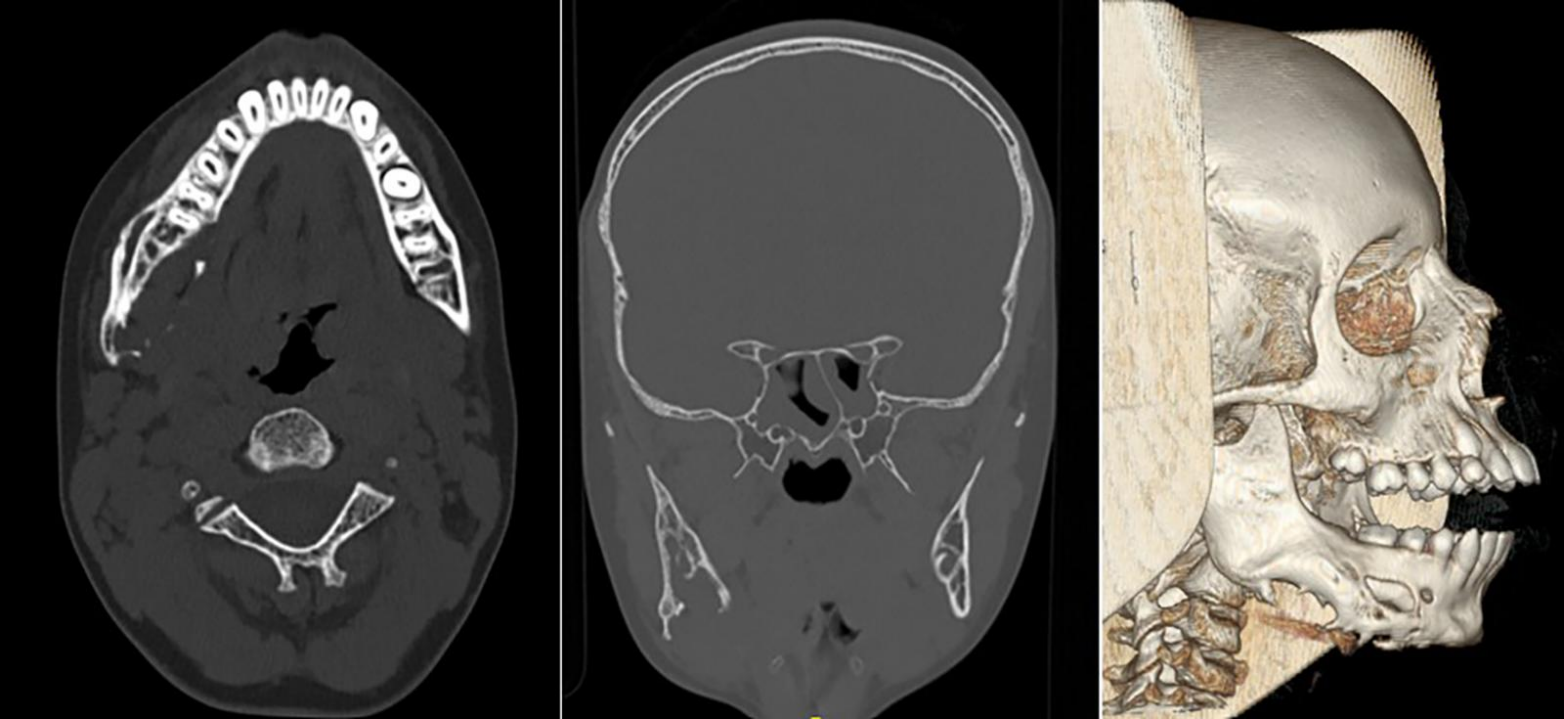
Computed tomography (CT) evaluation of the mandibular lesion. Left: Axial CT image showing an expansile osteolytic lesion of the right mandible with cortical thinning and focal lingual perforation. Middle: Coronal CT image demonstrating vertical extension of the lesion, replacement of normal trabecular bone, and involvement from the para-symphysis to the ascending ramus. Right: 3D reconstructed CT view highlighting the extent of mandibular destruction and external cortical deformation caused by the lesion.
